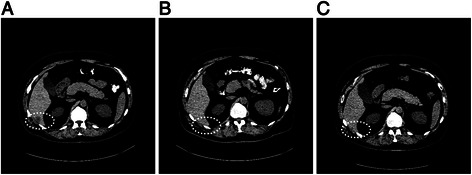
Computed tomography scans. A) at baseline, B) 2 months, and, C) 3.8 months after treatment initiation with a combination of a MEK and BRAF inhibitor, of a patient (case #8) with short-lived CR (TTF = 5.6 months), who demonstrated an *NRAS* mutation in a biopsy obtained 3.8 months after treatment initiation. At 2 months, the liver lesion has regressed; at 3.8 months it recurred.
